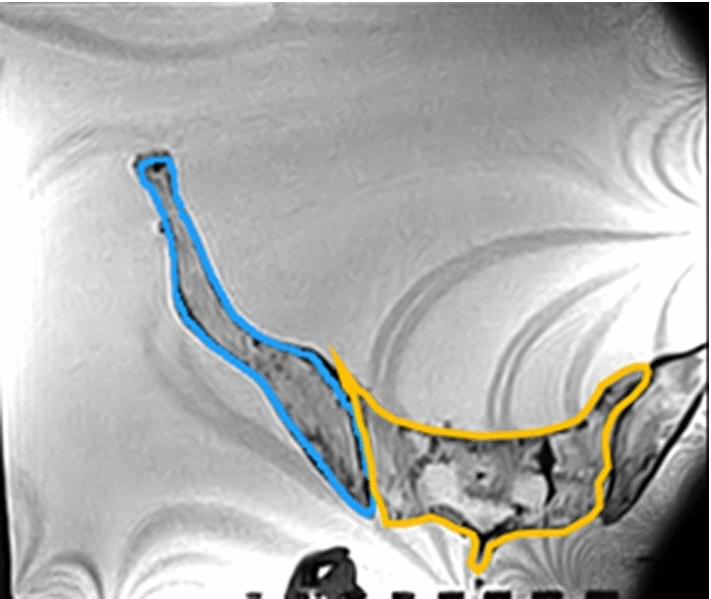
The labels of the sacrum and ilium on axial view of MRI. Blue line and orange line indicate left ilium and sacrum, respectively
